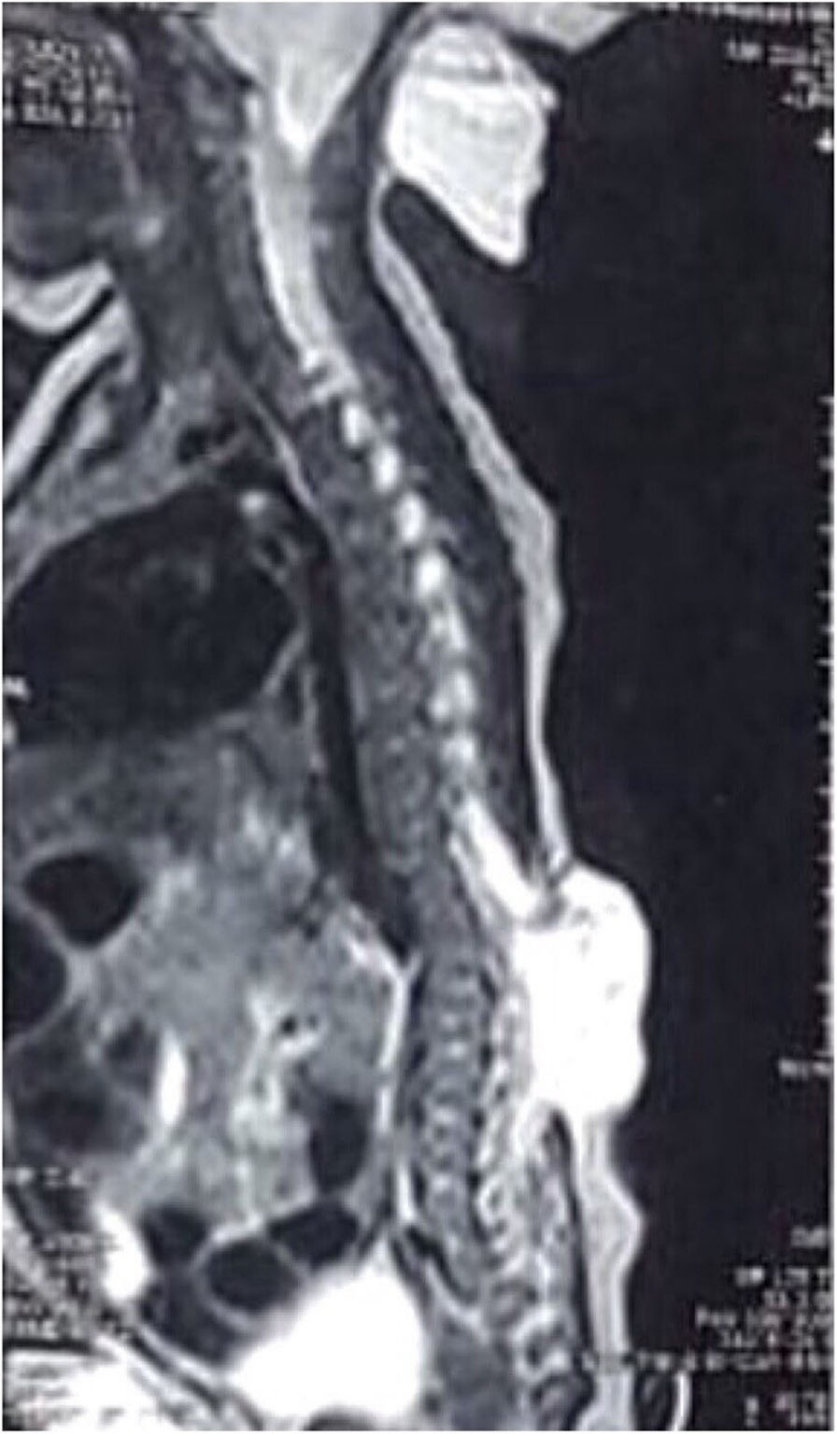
MRI Thoracolumbar spine showing CSF containing sac with neural tissue herniating through a defect in posterior elements of D11 to L1 vertebrae suggestive of thoracolumbar meningomyelocele with syrinx formation in proximal thoracic cord
